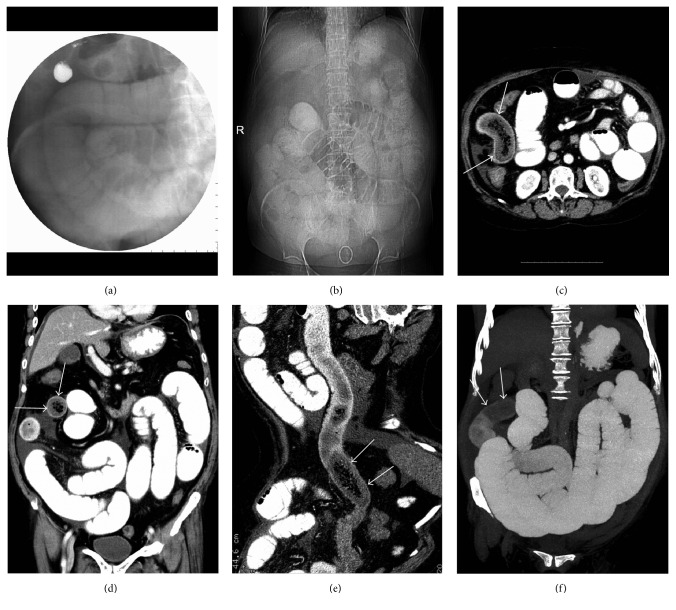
Phytobezoar in the middle segment of the small bowels causing SBO. (a) Gastrointestinal contrast radiography detected dilatation and fluid collection in the ileum. (b) Supine abdominal radiograph 24 hours after oral angiografin demonstration demonstrated no contrast agent accumulation in the colon which indicates a complete SBO. (c, d) MDCT axial and coronal images of the abdomen revealed a phytobezoar (white arrows) in the middle segment of the ileum and proximal distended bowels with contrast agent filling. (e) MDCT images with CPR clearly show the location of phytobezoar (arrows), proximal distended, and distal collapsed bowels. (f) MDCT with MIP image reveals a filling defect (arrows) at the obstruction site of the ileum and proximal distended small bowels.
